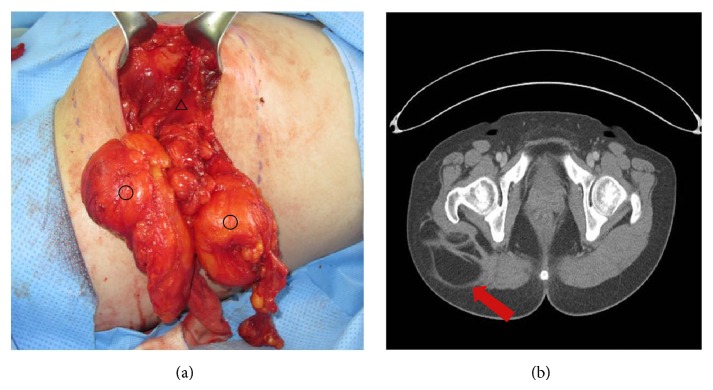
Case 12. (a) Intraoperative clinical photography. (○: intramuscular lipoma, △: gluteus maximus muscle). (b) CT image shows a 6.5 × 11.0 cm sized infiltrating lipomatous mass in the Rt. gluteus maximus muscle.
